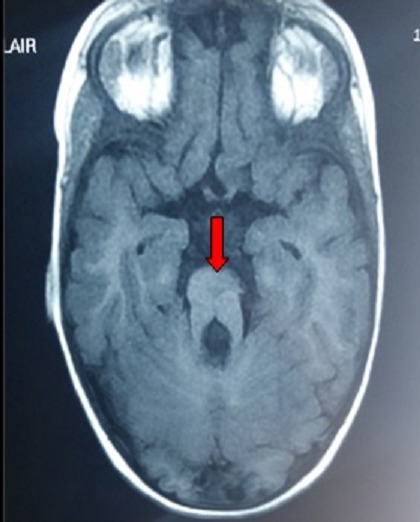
Cerebral MRI showing agenesis of cerebellar vermis causing the “molar tooth sign”
